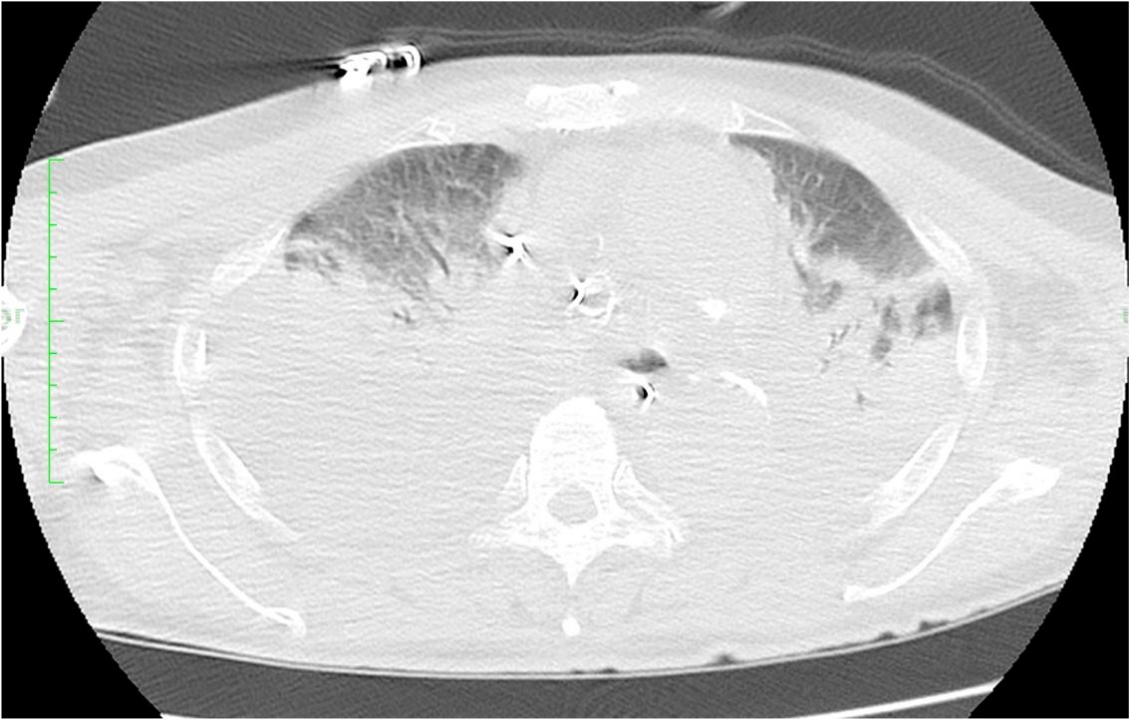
Massive bilateral consolidation was revealed by chest computed tomography.
